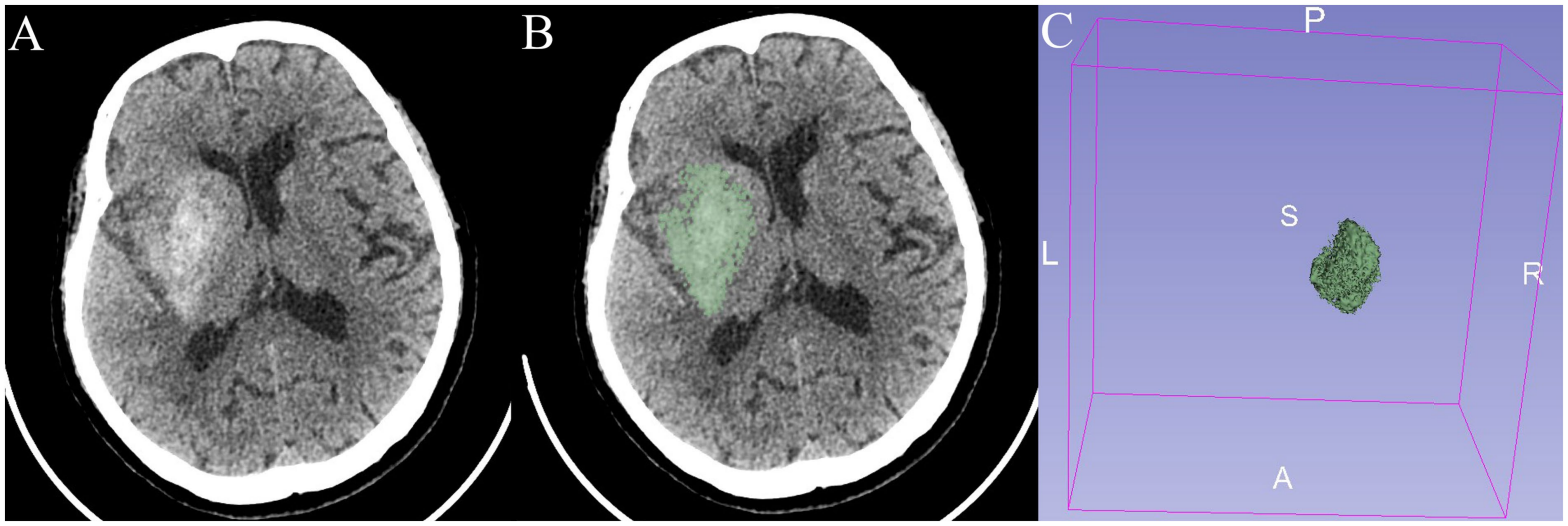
An 82-year-old patient presented with a right-sided cerebral ischemic stroke. (A,B) Non-contrast CT immediately after EVT shows contrast enhancement (CE). (C) The three-dimensional structure of CE in the brain is reconstructed with a volume of 24.9 mL.
